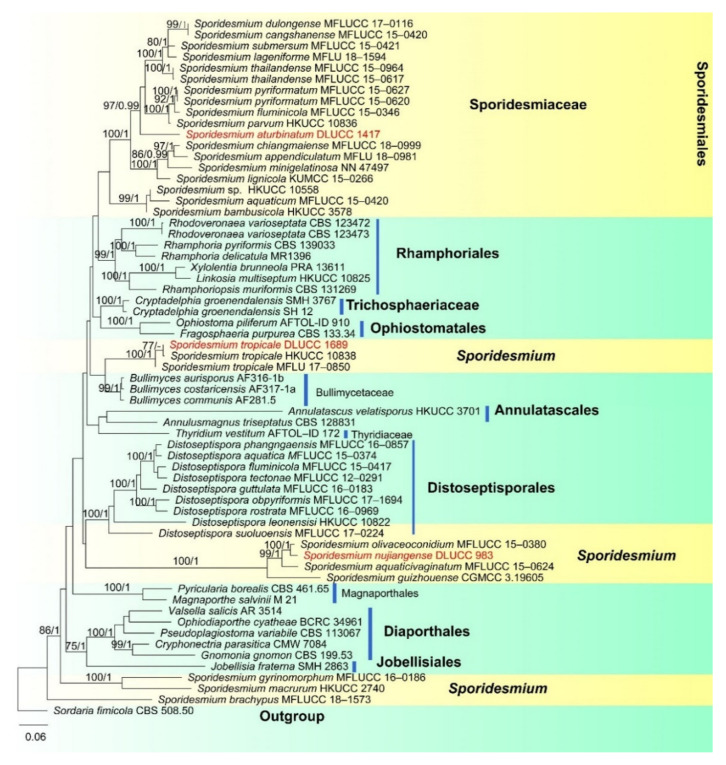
RAxML tree based on analysis of combined LSU, SSU, ITS TEF1-a and RPB2 dataset. The combined analyses include 62 strains with 4832 characters including gaps (LSU: 967 bp, SSU: 1235 bp, ITS: 624 bp TEF1-a: 935 bp and RPB2: 1071 bp). The tree is rooted to *Sordaria fimicola* (CBS 508 50). Tree topology of the maximum likelihood analysis and Bayesian analysis are similar. The RAxML analysis of the combined dataset yielded a best scoring tree with a final ML likelihood value of −44,590.479128. The matrix had 2439 distinct alignment patterns, with 50.13% undetermined characters or gaps. Estimated base frequencies were as follows: A = 0.244673, C = 0.255940, G = 0.282757, T = 0.216629; substitution rates AC = 1.228225, AG = 2.563347, AT = 1.222728, CG = 1.236937, CT = 5.801299, GT = 1.000000; gamma distribution shape parameter α = 0.277316. Bootstrap values for ML ≥ 75% and Bayesian posterior probabilities (PP) ≥ 0.95 are labelled on the nodes. The newly obtained sequences are indicated in red.
